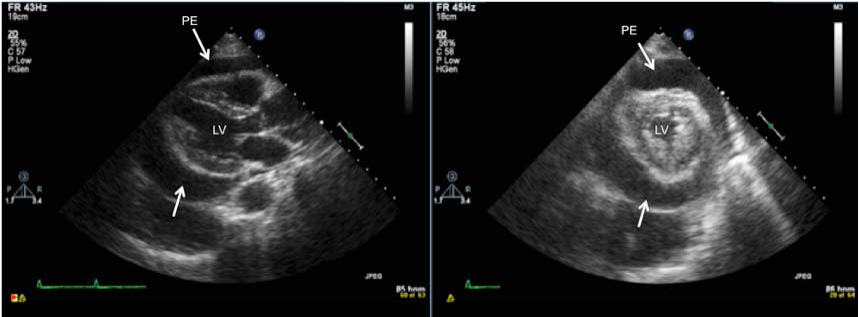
A transthoracic 2-dimensional echocardiogram. Note the circumferential pericardial effusion on the long- and short-axis parasternal views on this transthoracic echocardiogram, prior to pericardiocentesis. PE, pericardial effusion; LV, left ventricle
